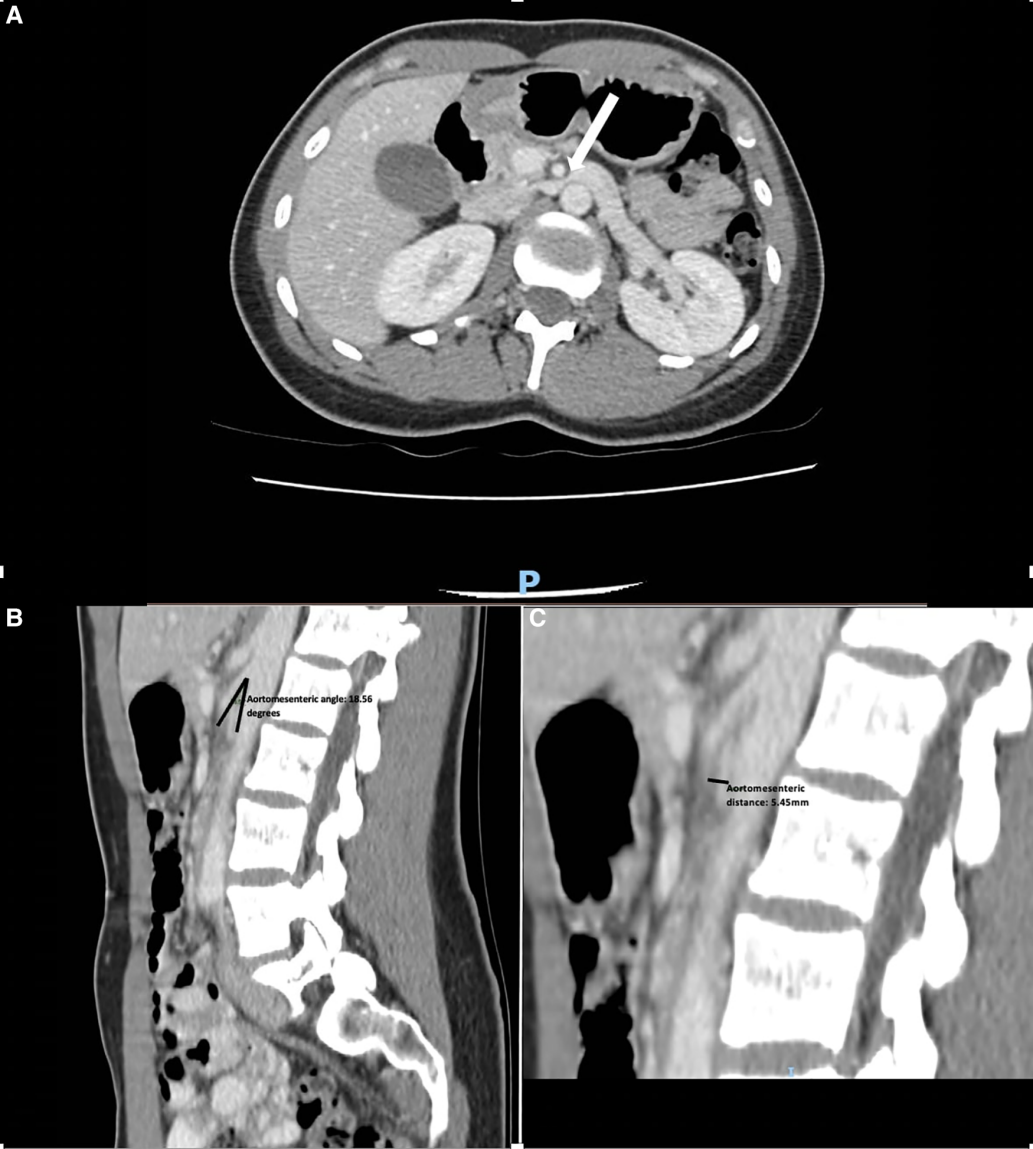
(A) CT scan demonstrating dilated left renal vein compressed between the superior mesenteric artery and aorta with an arrow pointing to the area of compression. (B) A sagittal CT scan demonstrating an aortomesenteric angle of 18.56°. (C) A sagittal CT scan demonstrating an aortomesenteric distance of 5.45 mm.
